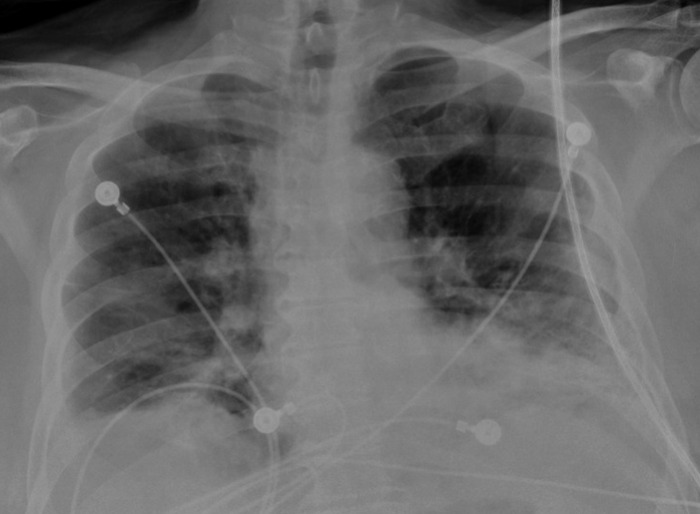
Admission chest x-ray was significant for multifocal bilateral pneumonia and a small left-sided pleural effusion.
